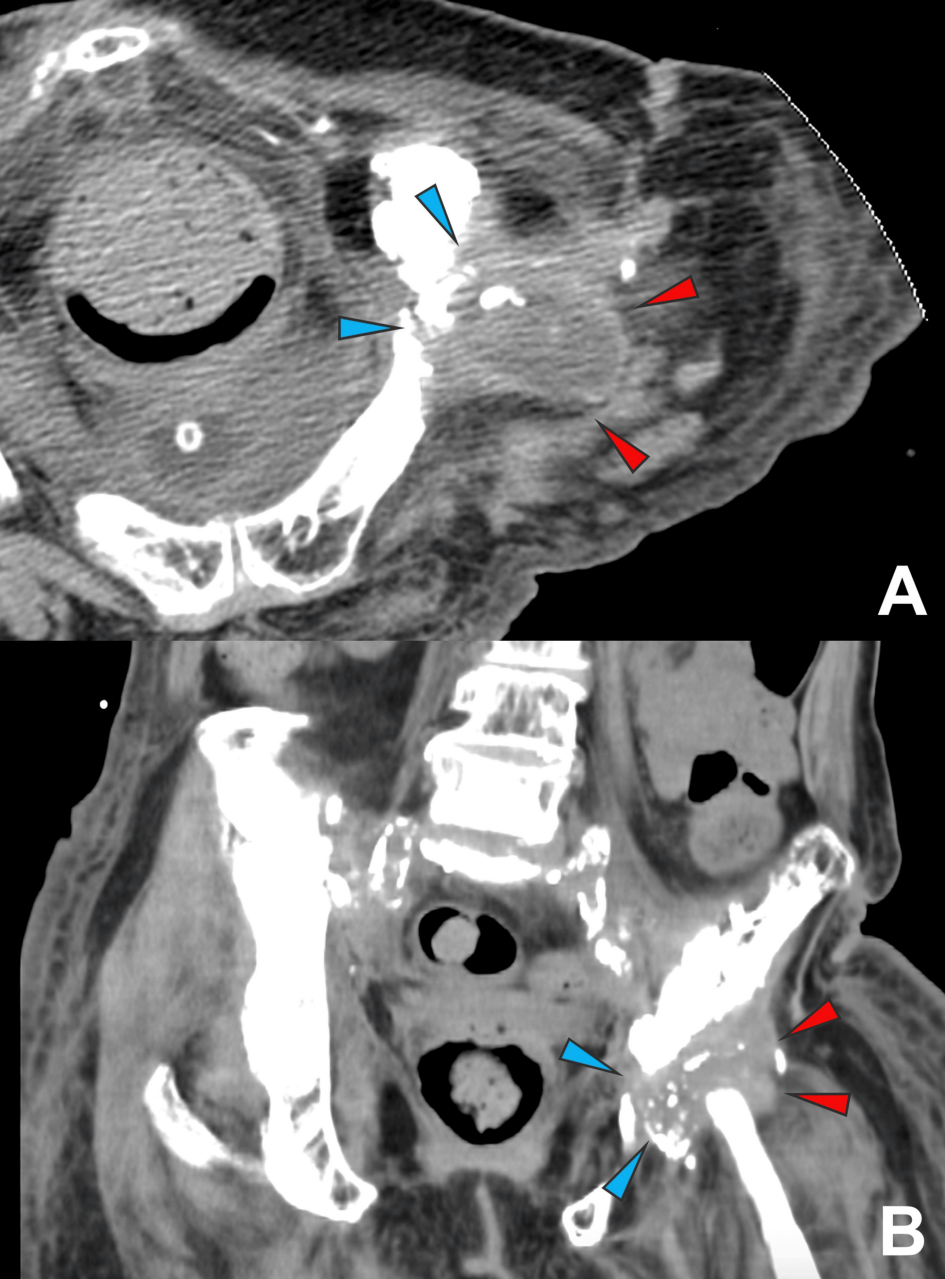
Contrast-enhanced lumbar CT images A, B: blue arrowheads indicate osteolytic changes in the left iliac bone, and red arrowheads indicate abscess formation.
